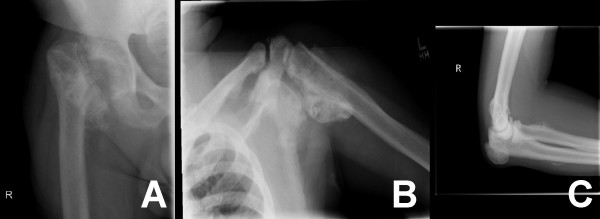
Radiographs of hetertopic bone formation and metaphyseal bone and appearance of sessile exostosis.
